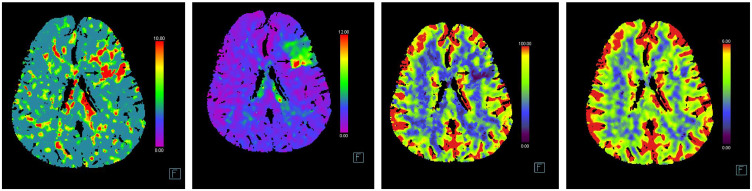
CT perfusion; from the left: Mean transit time (MTT), time-to-maximum (Tmax), cerebral blood flow (CBF), and cerebral blood volume (CBV), respectively. Study shows increased MTT, Tmax, and markedly decreased CBF, and normal CBV.
